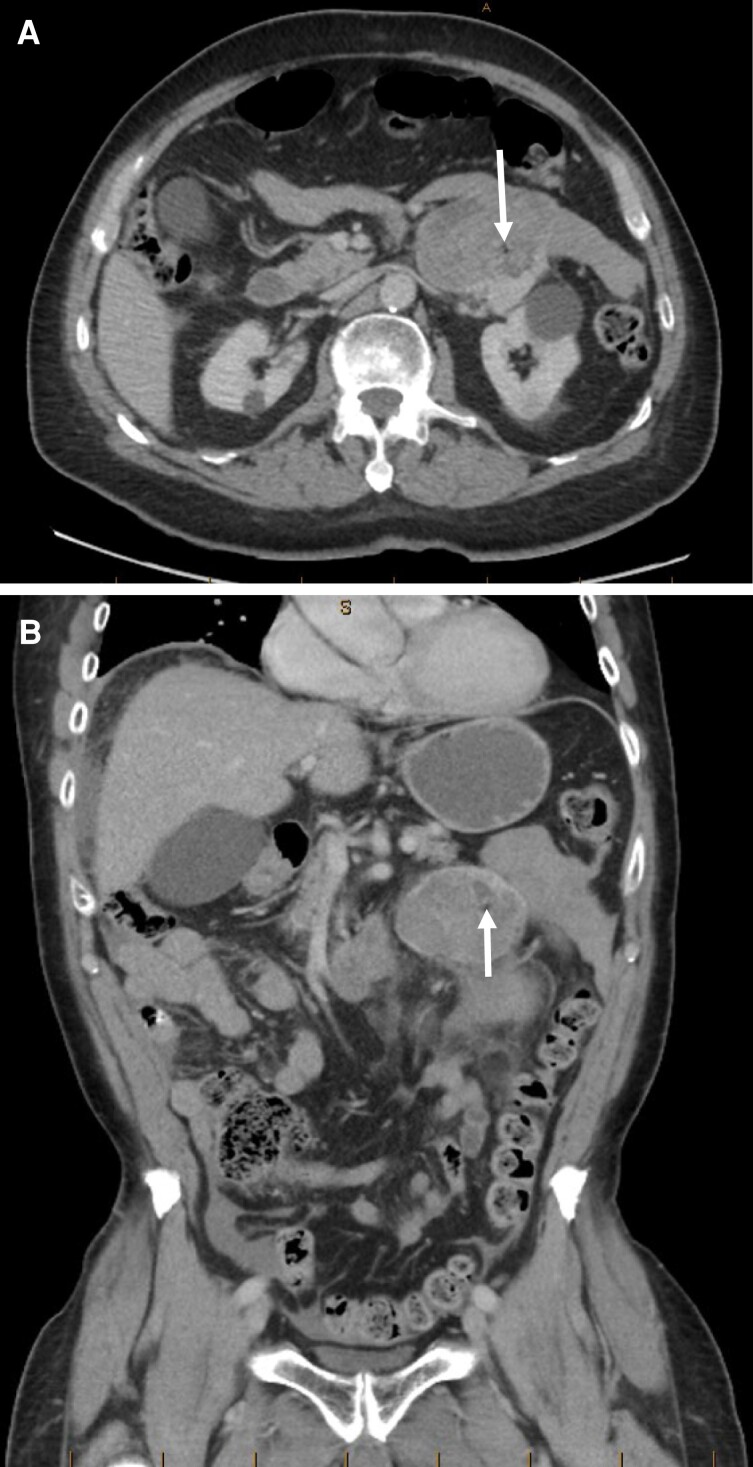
(A) Case 2, initial abdominal CT, axial portal venous phase. Focus of macroscopic fat indicated by arrow. (B) Case 2, initial abdominal CT, coronal portal venous phase. Focus of macroscopic fat indicated by arrow.
